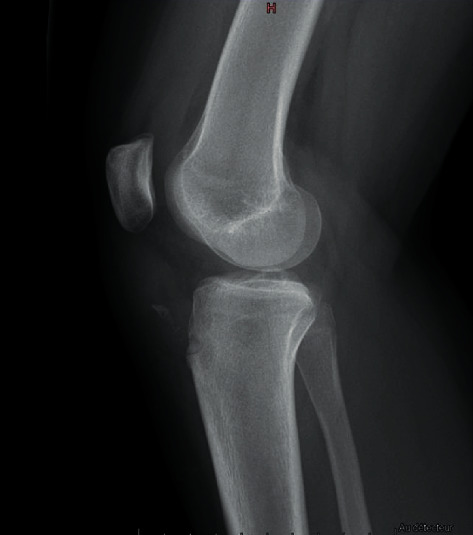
Lateral view radiograph revealed a distal patellar tendon avulsion with a slight patella alta.
